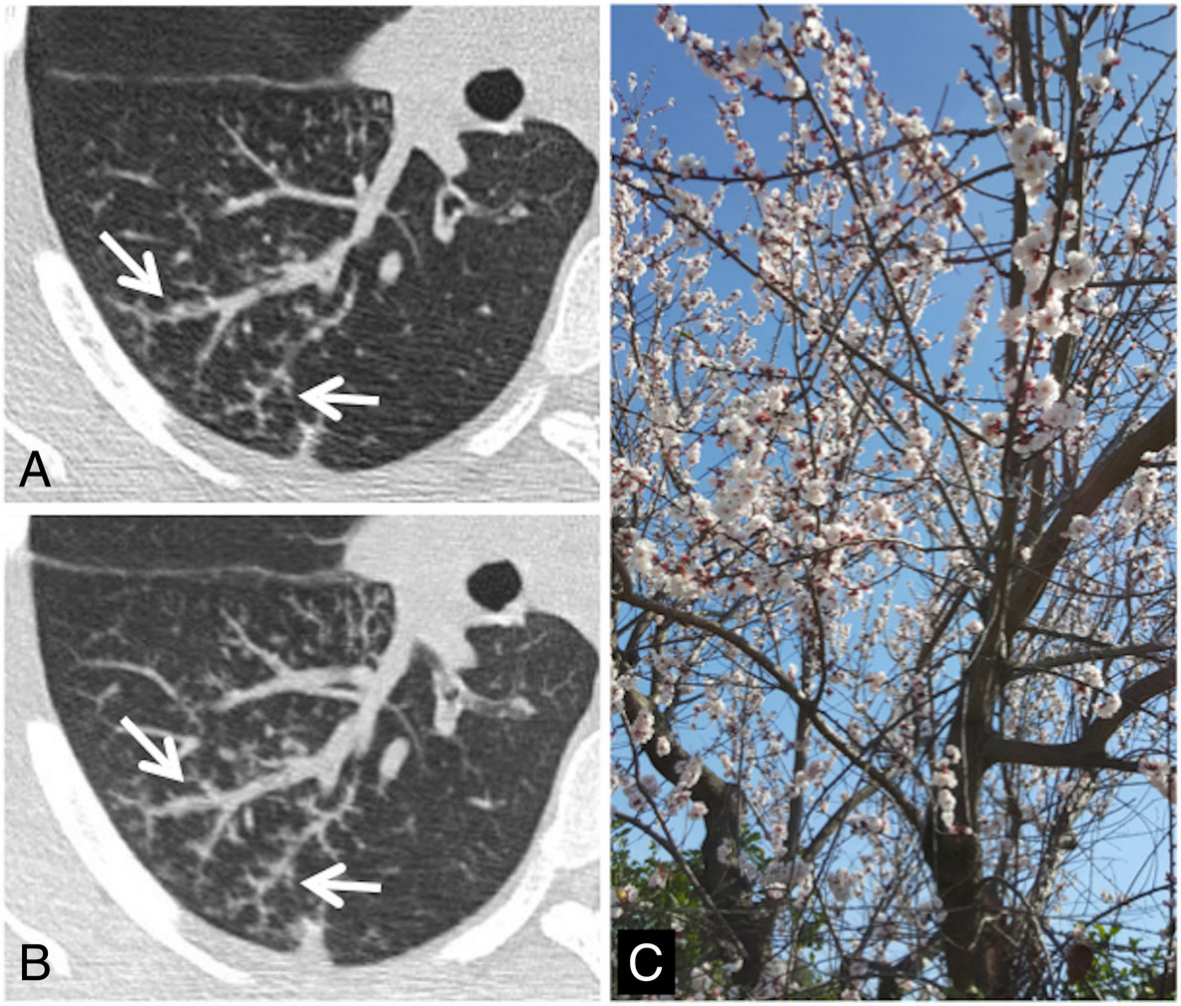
Tree-in bud sign. Small centrilobular nodularities and linear ramifications (white arrows in a and b) may be appreciated in the right lower lobe of a patient with cough and fever; these CT findings—which show the typical appearance of tree-in-bud—are related to the presence of inflammatory bronchiolitis. This tree-in-bud appearance is a morphological pattern that resembles a flowering tree (c)
